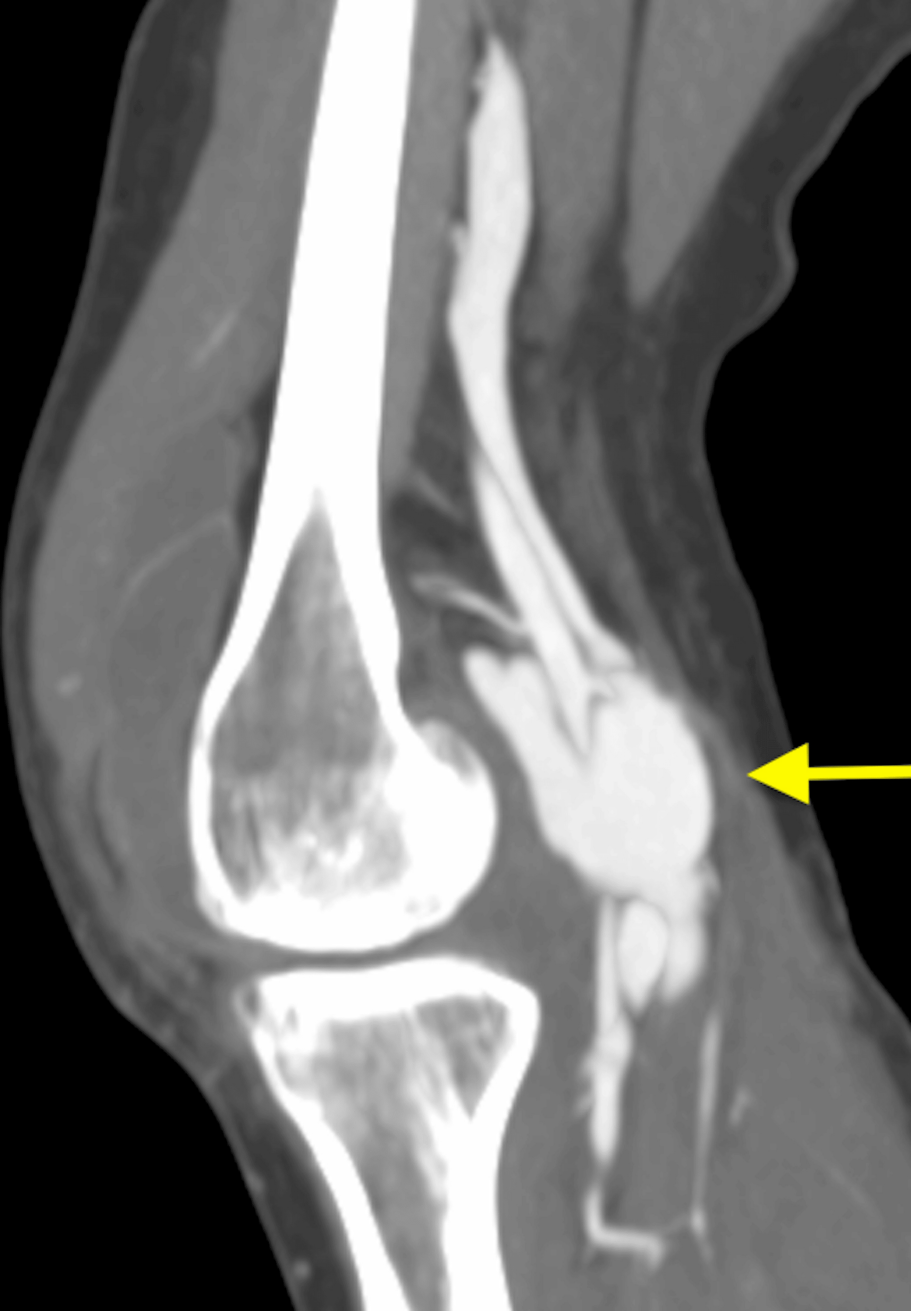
Computed tomography angiogram of the left knee with contrast (sagittal view) showing popliteal vein dilation (yellow arrow).
